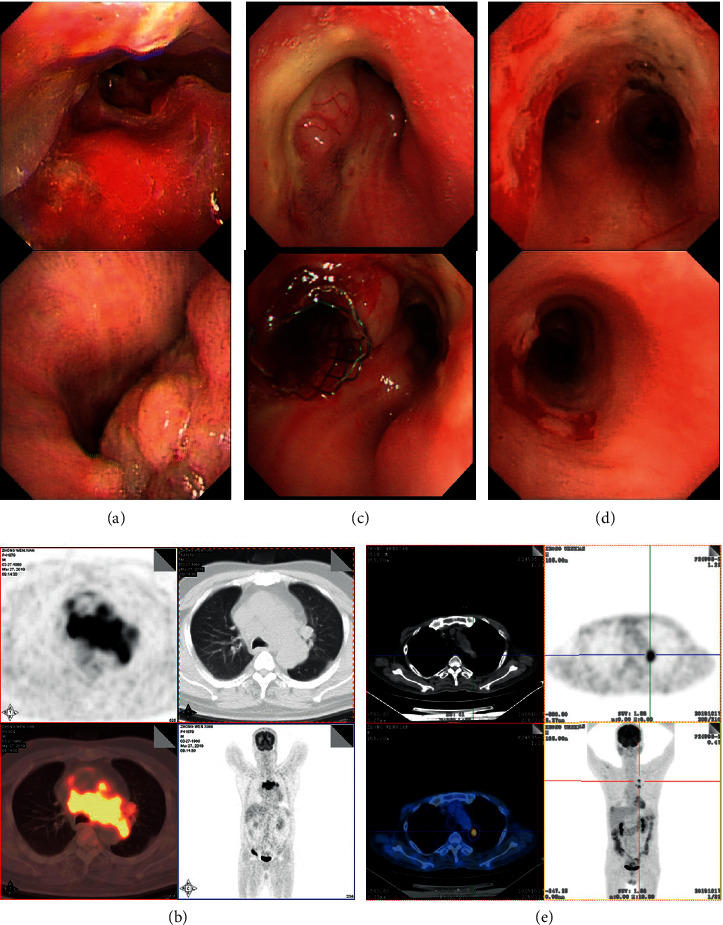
Fiberoptic bronchoscopy and PET/CT images. (a) Fiberoptic bronchoscopy revealed intratracheal mass in right main bronchi with complete right mainstem bronchus occlusion before treatment. (b) PET/CT image showing left hilum and mediastinal mass (41 × 87 × 76 mm, FDG uptake 20.6) with compression of the pulmonary artery, lower tracheal segment, bilateral main bronchi, and superior vena cava. (c) Right mainstem bronchus was completely occluded, and a tracheal stent was implanted. (d) Bronchus occlusion was significantly improved after treatment with PD-1 inhibitor and DNMTi/HDACi. (e) Restaging PET image showing dramatically shrinkage of hilum and mediastinal mass (16 × 15 mm, FDG uptake 8.0) after a triple combination treatment of DNMTi/HDACi plus PD-1 inhibitor.
